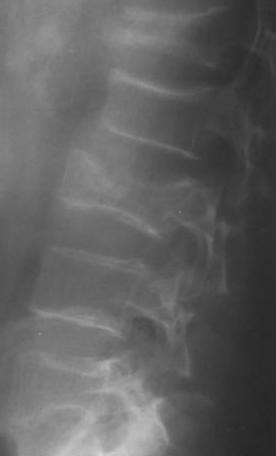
Radiography of lumbar vertebras after seizures showing fractures (patient n 2).
